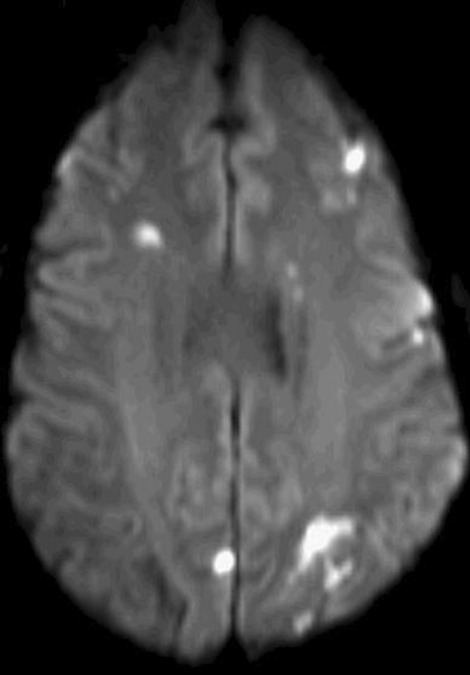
Case 3. Diffusion weighted brain MRI showing multifocal infarctions consistent with embolism
